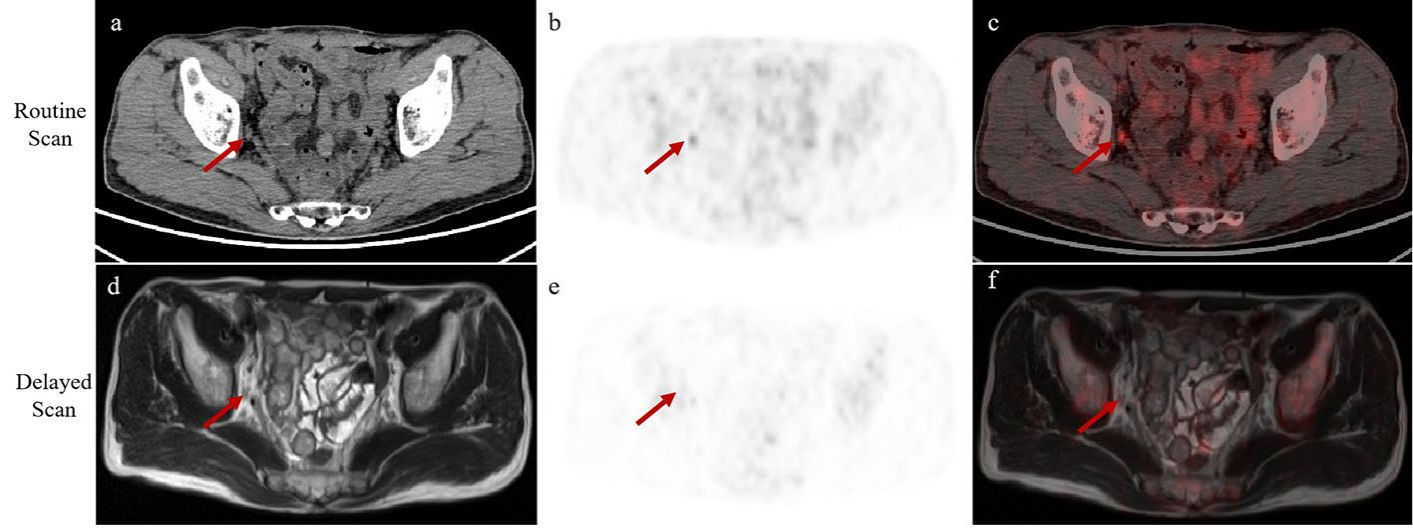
A 63-year-old man with prostate cancer, GS 4 + 4 and serum PSA value of 11.68 ng/mL. In routine imaging, performed 50 min after [^18^F]AlF-PSMA-11 injection, (a) axial PET/CT, (b) PET and (c) fused PET/CT images show tracer uptake (SUV_max_, 5.3) on the right pelvic wall (red arrows). In delayed imaging, performed 120 min after [^18^F]AlF-PSMA-11 injection, (d) PET/MR-T1WI, (e) PET and (f) fused PET/MR images show no tracer uptake in the lymph nodes (red arrows). The final surgical pathology confirms the absence of lymph node metastasis in this patient
